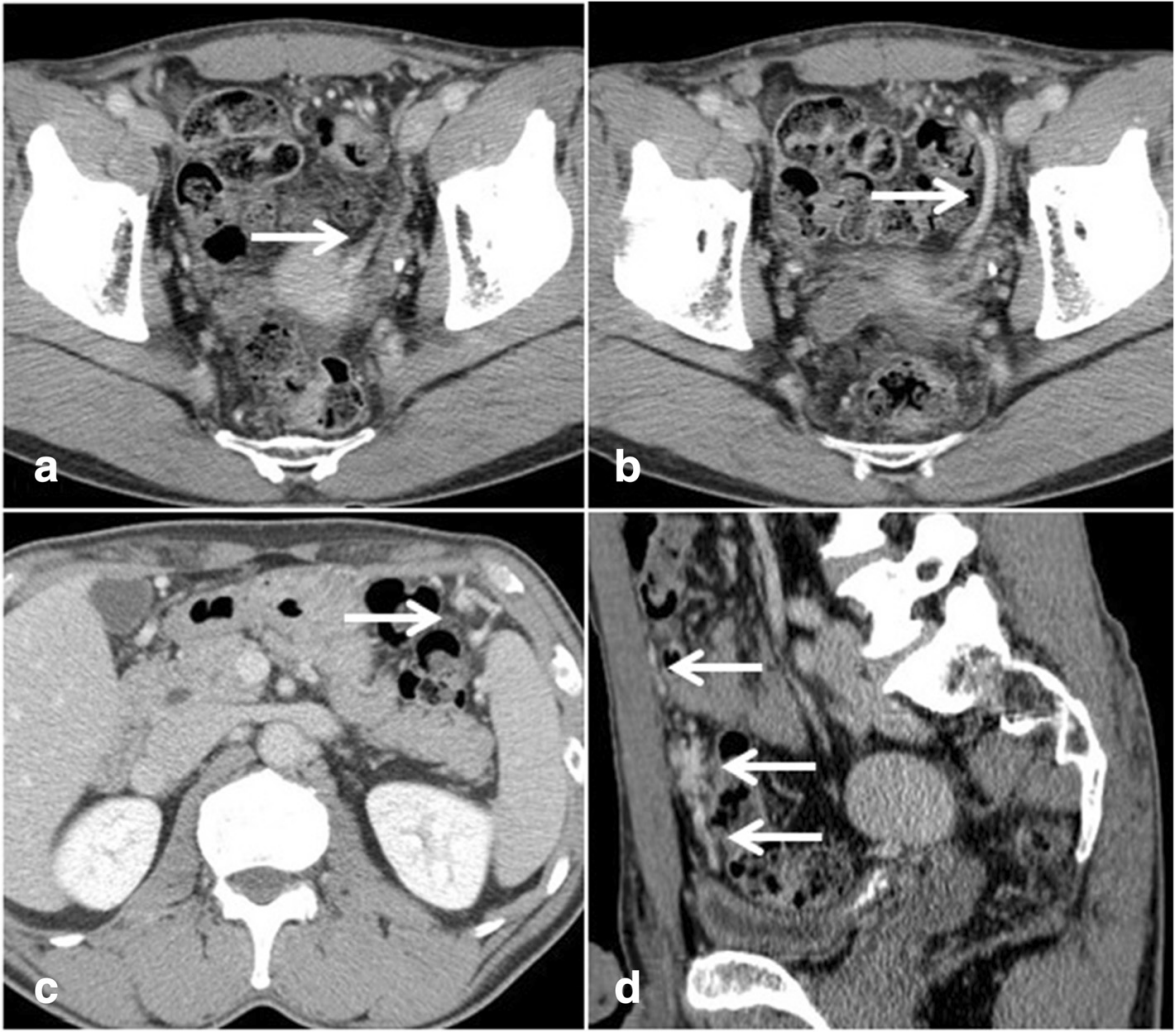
Preoperative computed tomography. Dynamic computed tomography shows the feeding blood vessel of the mass (a, *arrow*), which extended to the ventral side (b, *arrow*), and was derived from the left gastroepiploic artery (c, *arrow*). Coronal image shows that the vessel ascended toward the ventral side (d, *arrows*; coronal image)
